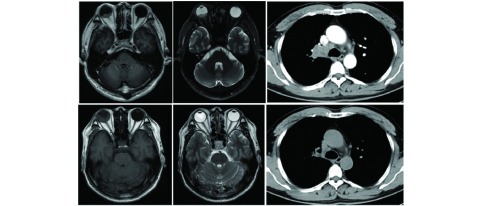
NO.4患者影像学结果：吉非替尼及眼部放疗治疗前后头颅核磁及胸部CT变化。基线：头颅核磁示右眼底稍短T1短T2信号（A和B）；胸部增强CT右上叶开口占位，纵膈淋巴结增大（C）。治疗后（吉非替尼及眼部局部放疗）：患者眼部疼痛缓解，右眼底病变的大小和信号均有变化（D和E）；右肺病变较前缩小（F）。 Imaging findings of patient NO. 4: changes of brain magnetic resonance (MRI) imaging and chest computed tomography (CT) before and after gefitinib treatment and local (ocular) treatment. Initial presentation: Brain MRI images at the initial presentation revealed a mass in the choroid of the right eye (A and B). Chest computed tomography scan at the initial presentation revealed a mass in the upper lobe of the right lung with mediastinal lymphadenopathy (C). After treatment (gefitinib treatment and radiation therapy): Brain MRI images after treatment revealed the choroidal metastasis changed in shape and signal (D and E), with the patient's eye aching improving. Chest computed tomography scan after treatment revealed the shrinking of the tumor in the right lung (F).
